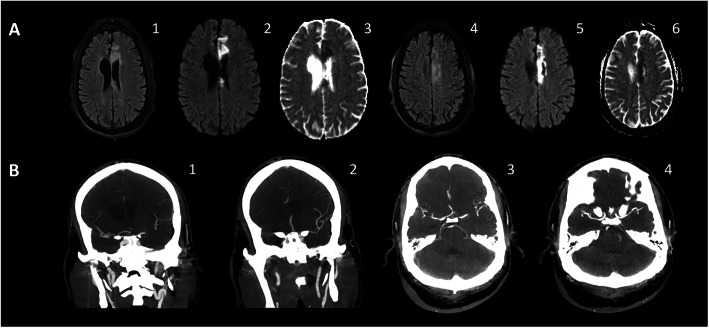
Inflammatory vasculopathy. A 45-year-old SARS-CoV-2-positive man presented chiefly with encephalopathy and aphasia, without concurrent respiratory or any other systemic symptoms. Serum levels of inflammatory makers (CRP; 152.4 mg/dl, ESR; 91 mm/h) and D-Dimer (464 mg/dl) were elevated. Anticardiolipin IgM antibodies were 52.3 (normal range 0–12.5). Past medical history is notable for diabetes and hypertension. Axial FLAIR (1, 4) MR images show gyriform signal hyperintensity of the left paramedian frontal lobe and genu of the corpus callosum with corresponding high signal intensity on DWI (2, 5) and low signal intensity on ADC (3, 6) compatible with an acute ischemia. The maximum intensity projection (MIP) of CT angiogram demonstrates irregular areas of narrowing of the left A1 and M1 segments. These findings are consistent with inflammatory vasculopathy, likely related to the interplay among systemic inflammation, immune dysregulation, and anticardiolipin antibodies. Direct infection of the endothelial cells and its contribution to the vascular irregularity of the affected vascular segments cannot be excluded. The patient received tocilizumab 8 mg/kg intravenously and a 5-day course of intravenous pulse methylprednisolone (1000 mg/day). He was discharged to a subacute rehabilitation facility with mild improvement of aphasia and cognitive deficit
